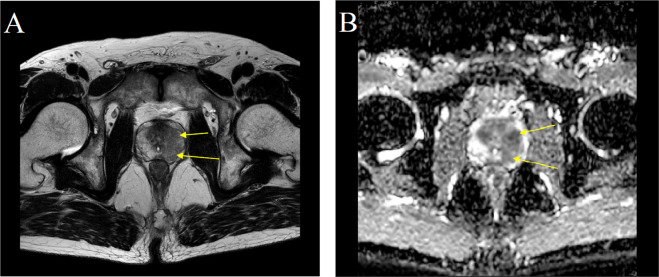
mpMRI of a male patient with stage T1 high-grade bladder cancer 3 months after intravesical BCG. (A) T2-weighted MRI demonstrating focal circumscribed nodules of decreased signal involving the transitional zone and left peripheral zone (arrows). (B) Apparent diffusion coefficient map demonstrating a decreased signal corresponding to the T2-weighted images (arrows).
